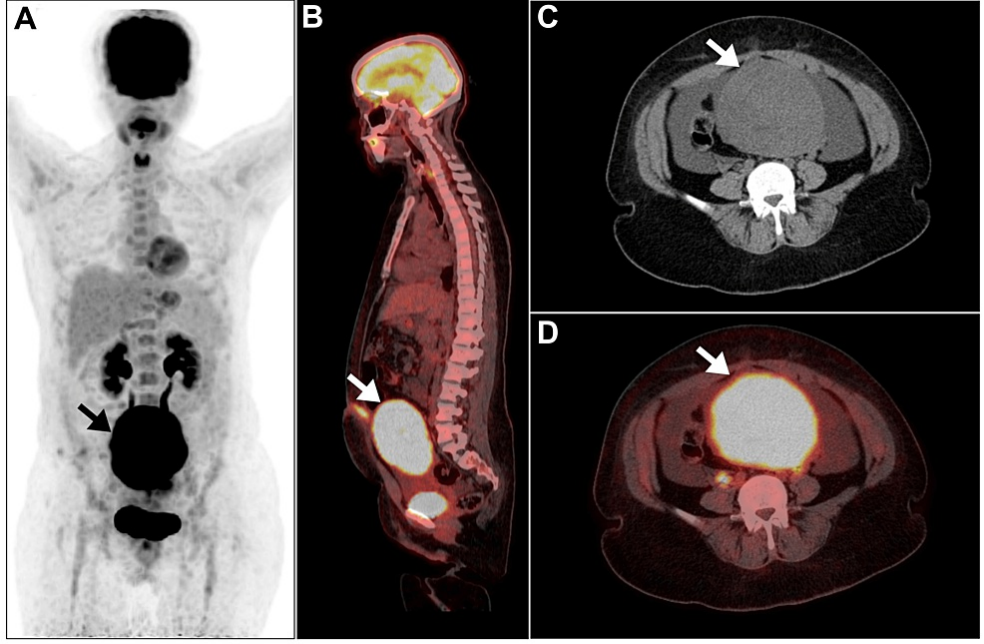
[18F]FDG PET/CT image. (A) MIP revealing evidence of a large hypermetabolic uterine mass (arrow). (B) Sagittal fused [^18^F]FDG PET/CT image demonstrating high hypermetabolic activity involving the enlarged uterus and extending to the umbilicus, with a maximum SUVmax of 26.3 (arrow). (C) Axial CT image and (D) axial PET/CT image demonstrating massive uterine enlargement with intense [^18^F]FDG activity (arrow). [^18^F]FDG: [^18^F]fluorodeoxyglucose; PET: positron emission tomography; CT: computed tomography; MIP: maximum intensity projection; SUVmax: maximum standardized uptake value
